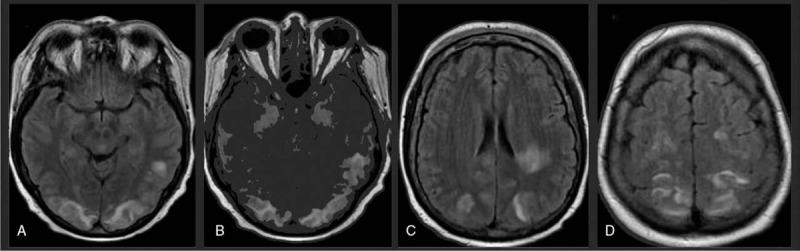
Case 3, MRI showed parieto-occipital hyperintensities on FLAIR (A–D). These were completely resolved a few days after treatment.
